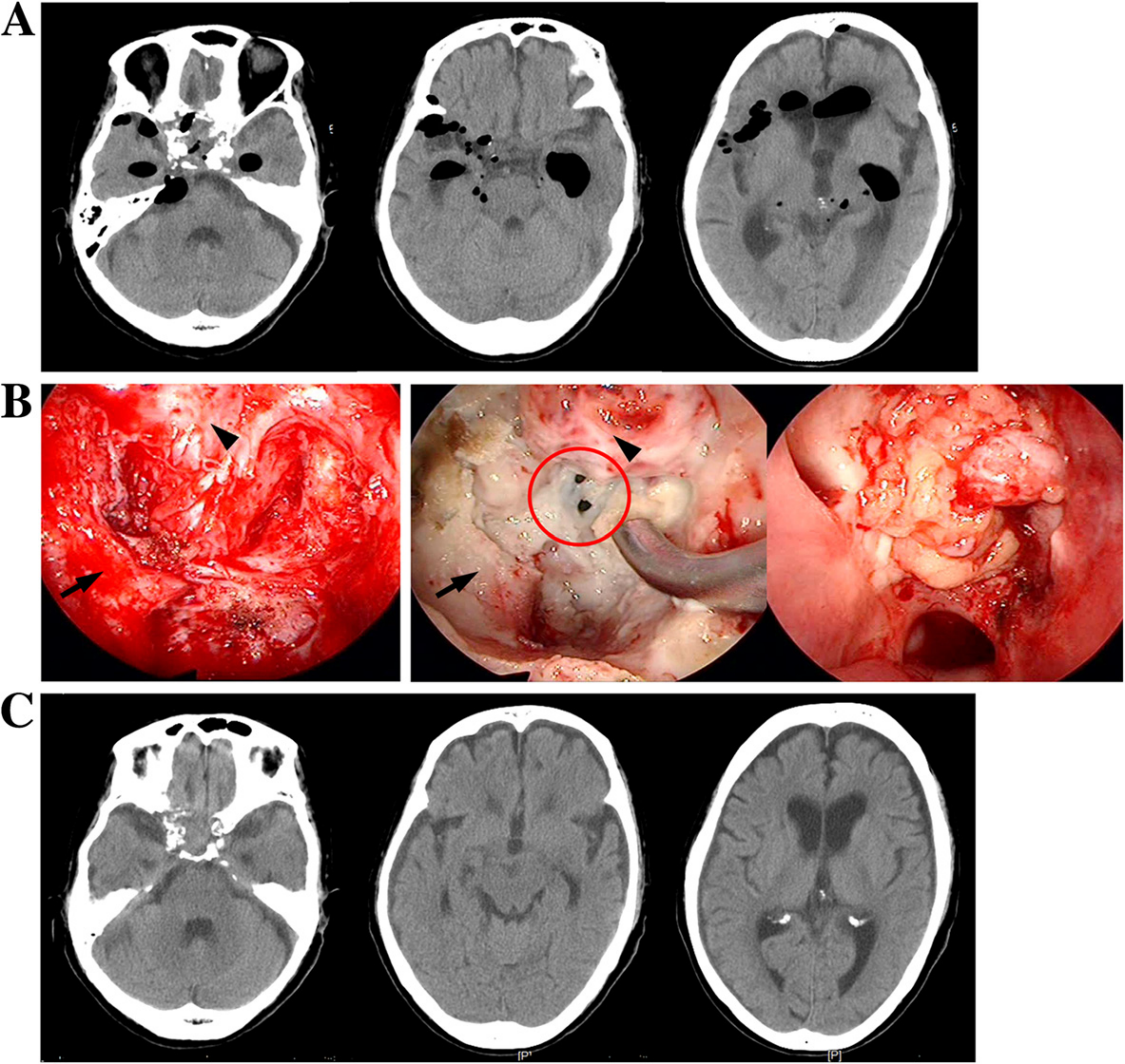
A. Computed tomography 6 months after CyberKnife^® ^treatment. Multiple extremely low density areas are shown in subarachnoid spaces and lateral ventricles, suggesting pneumocephalus. B. Endoscopic repair of cerebrospinal fluid (CSF) leakage. Left: The endoscopic appearance of subtotal tumor resection in the first surgery. Center: Endoscopic observation of the residual tumor 6 months after CyberKnife^®^ treatment. All irradiated tissue is hypovascular and whitish, and two small holes are identified in the dura mater at the bottom of sella turcica (red circle). Right: Fat tissue was used as a patch and fixed in place with fibrin glue. Black arrows show the lateral bone of the floor of the sella turcica and black arrowheads indicate residual tumor. C. Computed tomography after repair of CSF leakage. Air had completely disappeared 2 weeks after the endoscopic repair of CSF leakage.
